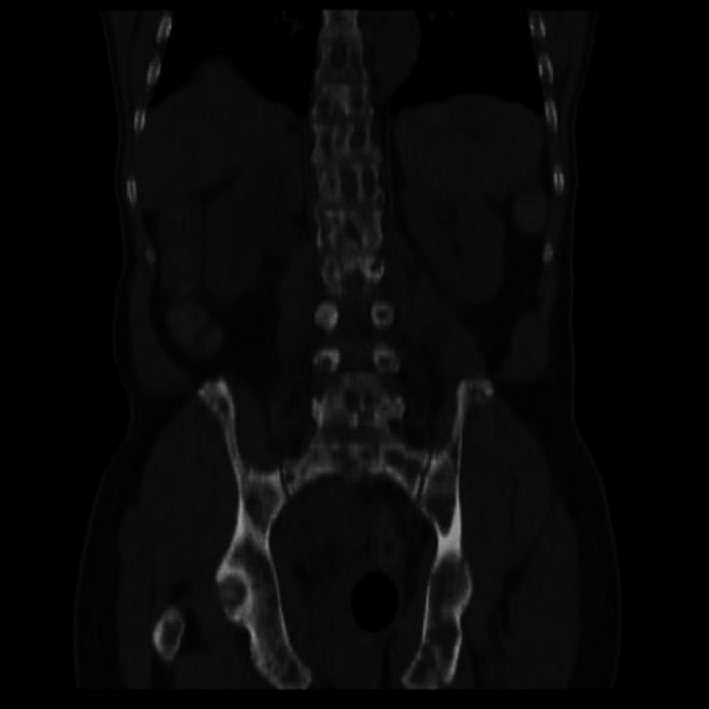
Coronal reformatted CT showing extensive lytic lesions/hypodensities on the demonstrated bones
